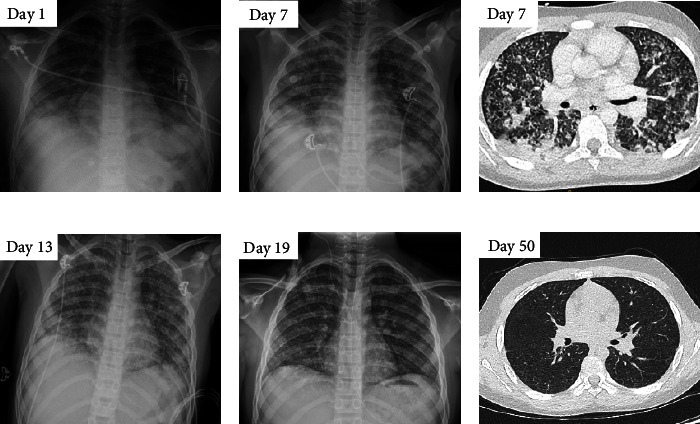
Pulmonary evolution on chest X-ray (CXR) and chest computer tomography (chest CT) during hospitalization and after discharge. (a) CXR on day 1. (b) CXR on day 7. (c) Chest CT on day 7. (d) CXR on day 13. (e) CXR on day 19. (f) Chest CT on day 50.
